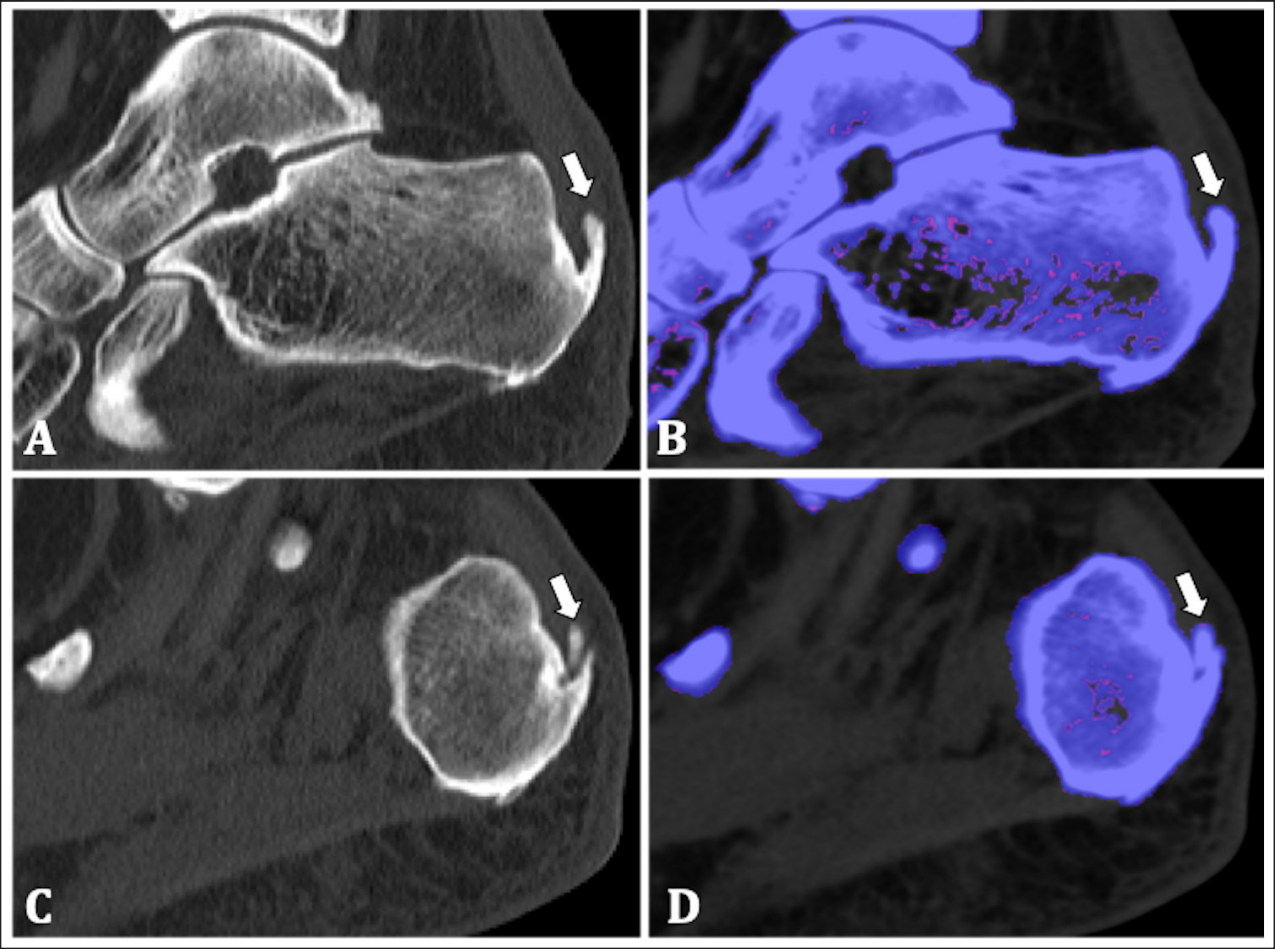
70-year-old woman. (A, C) Sagittal native CT image of the left and right calcaneum respectively show enthesophytes at the Achilles tendon insertions (arrows). (B, D) Post-processing shows there is no uric acid deposition (arrows).
